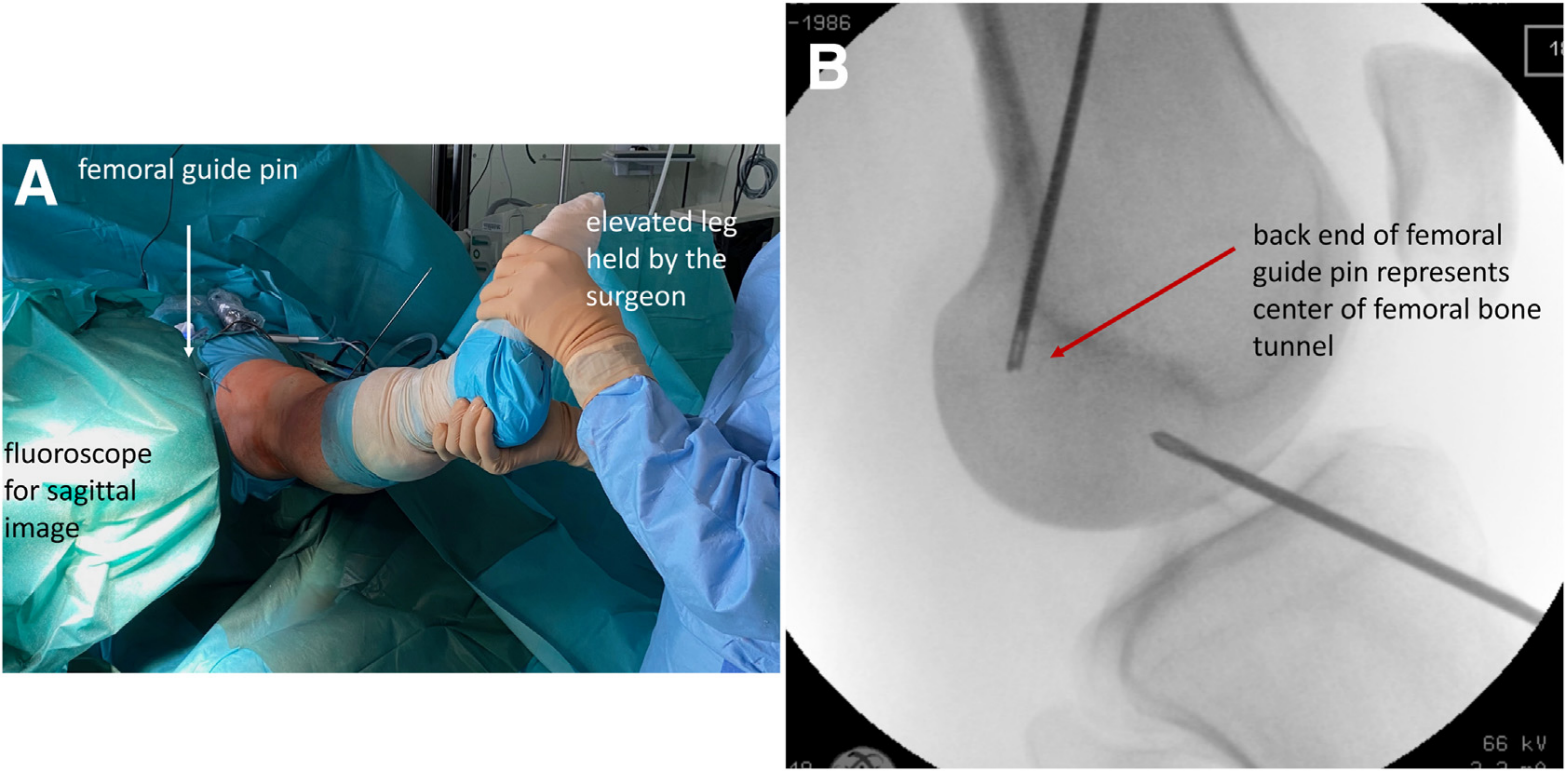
Technique of obtaining a true lateral radiograph with a guide pin in place and positioned so that the end of the pin is flush with the lateral notch cortex. (A) A technique of obtaining a true lateral radiograph in a right knee intraoperatively. The surgeon holds the elevated leg at the foot with the fluoroscope in sagittal orientation By sideway movements and rotation of the leg, a perfect alignment of both condyles can easily be achieved for a true lateral radiograph. (B) Example of a true lateral radiograph with both condyles in perfect alignment. The back end of the femoral guide pin represents the center of the femoral bone tunnel.
